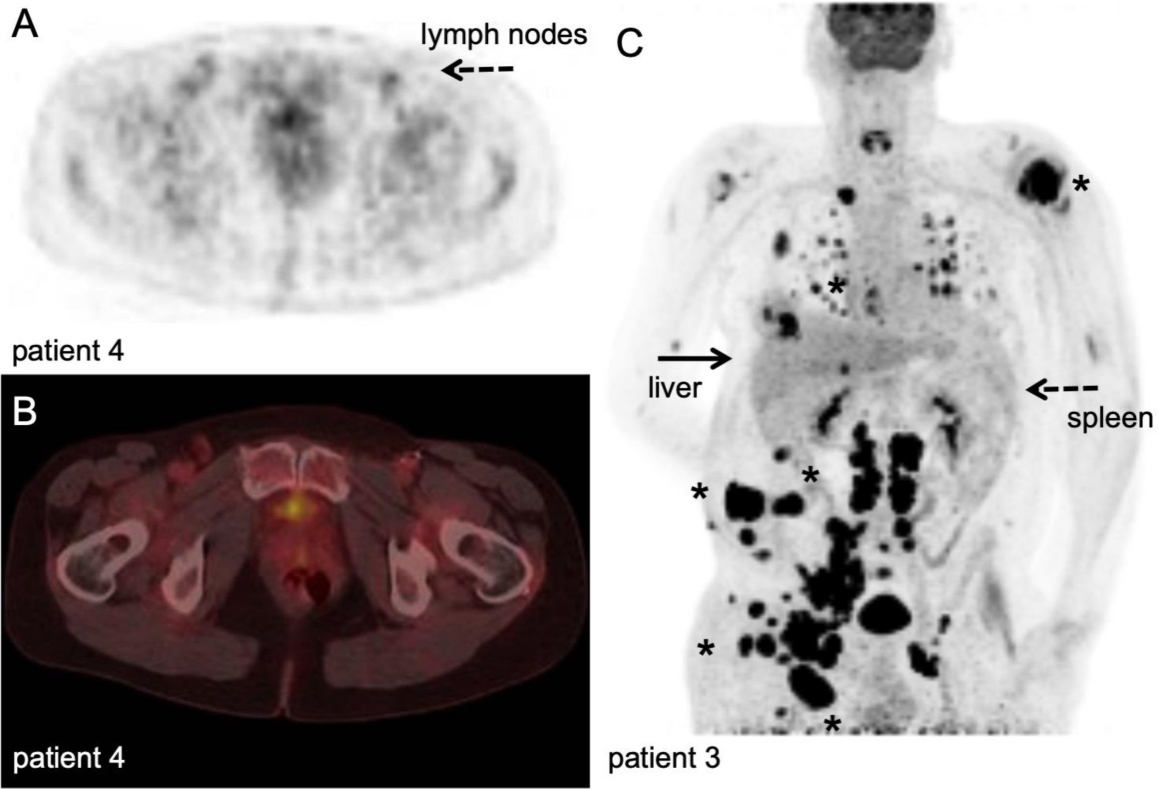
[^18^F]FDG PET/CT example. Stage III melanoma patient during adjuvant immune therapy, scanned at 60 minutes after IV injection of 207 MBq. A-B: transversal PET and fused PET/CT images; upon intranodal injection with antigen loaded dendritic cells, a faint signal increase in the injected and subsequent draining lymph nodes in the right inguinal region (dashed arrow) was observed which was not correlated to the magnitude of antigen specific T-cell responses. *(*78*, unpublished results).* C: Maximum intensity projection; *in vivo* biodistribution of [^18^F]FDG in the same stage IV metastatic melanoma patient as Figure 4 with multiple lesions in lymph nodes, subcutaneous tissue and lung (asterisk). Note the normal physiological activity as well as high uptake in the metastatic tumour lesions. *With courtesy of B. van der Hiel.*
